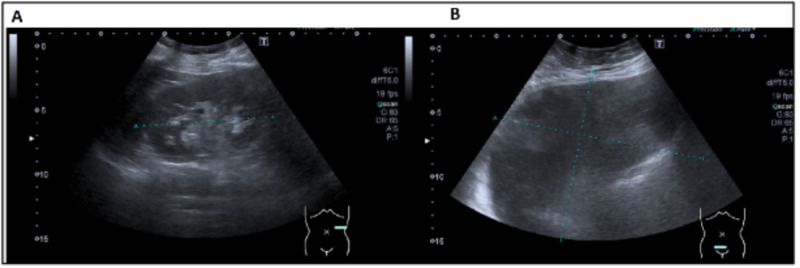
Transabdominal ultrasonography showing a transverse view of the left upper quadrant (A) and lower abdomen (B) of a cystic lesion.
